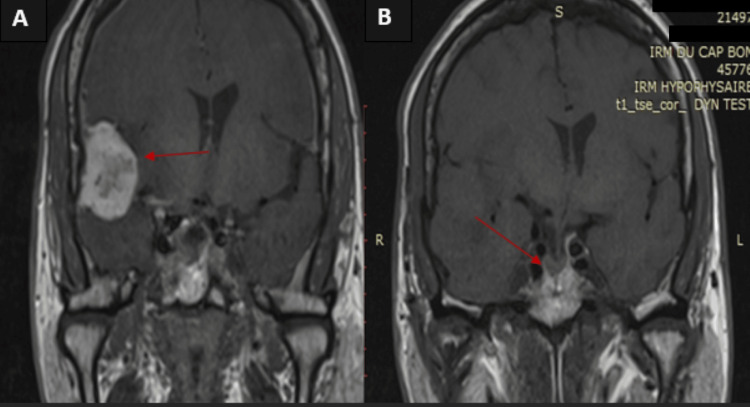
Hypothalamic–pituitary MRI of our patient Image 1A: Right temporoparietal extra-axial, dural-based mass consistent with meningioma, measuring 32 × 28 × 27 mm (red arrow), demonstrating significant mass effect with compression of the right lateral ventricle and associated leftward midline shift. Image 1B: Asymmetry of the pituitary gland, with increased thickness on the right, secondary to a mass effect on the midline, without any detectable adenoma (red arrow).
